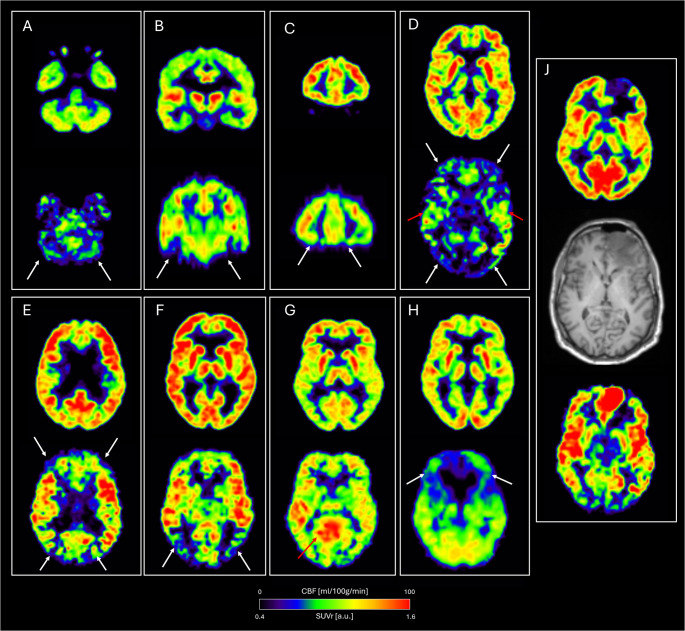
Examples of discordant ASL MRI CBF findings in healthy aged controls with normal [^18^F]FDG PET. For each example corresponding FDG PET (top) and ASL CBF (bottom) is shown using fixed scaling (as in Fig. 1). Arrows point to areas of apparent hypoperfusion (white) or hyperperfusion (red) with normal [^18^F]FDG uptake. A-C typical defects in basal structures due to susceptibility artefacts in EPI sequence (and not leading to rating as discordant), D global transit time artefacts causing widespread hypoperfusion and vascular high signal in insula, E-F symmetric hypoperfusion in watershed areas likely due to transit time effects, G hyperperfusion in posterior cingulate areas of unknown origin, H large area of pronounced hypoperfusion of unknown origin, J true high perfusion and low [^18^F]FDG uptake in large frontal meningioma found in healthy control subsequently excluded
